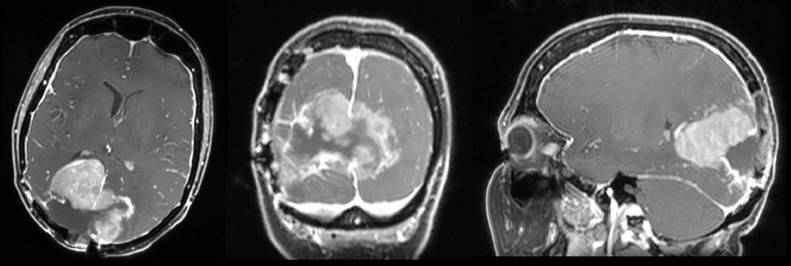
Magnetic resonance images of the brain in February 2020; axial, coronal, and sagittal image showing progression of disease of the anaplastic meningioma in both occipital lobes.
